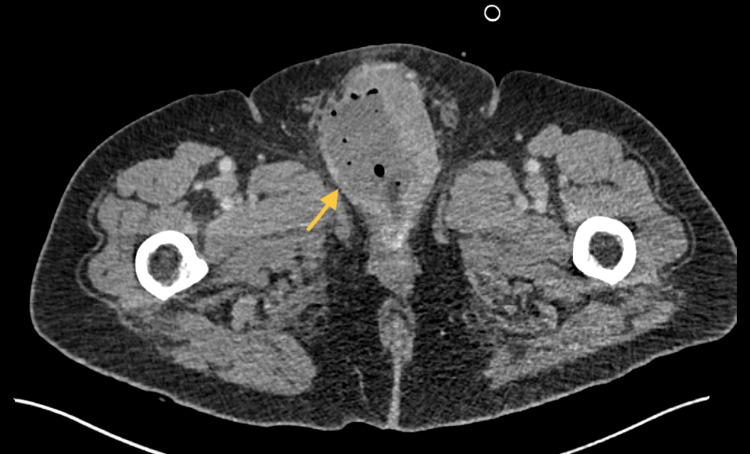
Initial CT scan demonstrating perineal inflammatory condition
